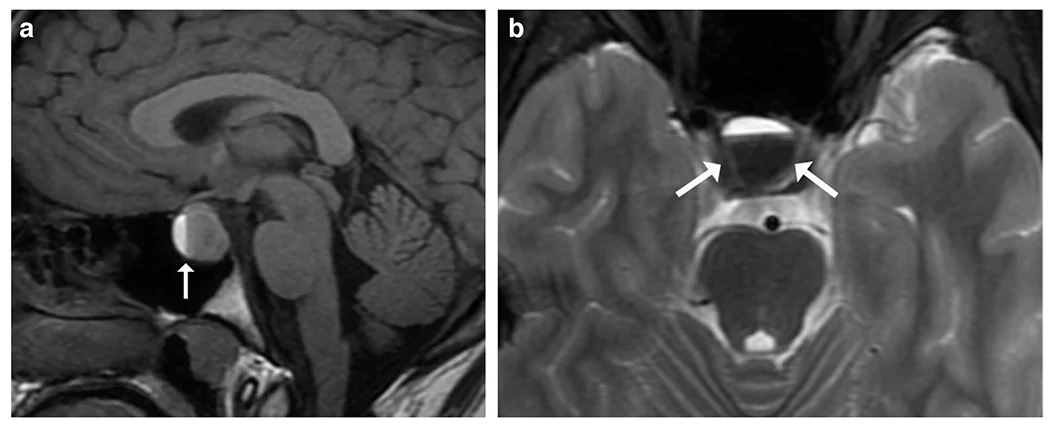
Macroadenoma in a 16-year-old female with elevated prolactin levels. a Sagittal T1-weighted sequence through the midline shows a rounded lesion in the sella containing a heme-fluid level (*arrow*) measuring 18 mm in diameter. b Axial T2-weighted image through the sella redemonstrates the lesion with a heme-fluid level
